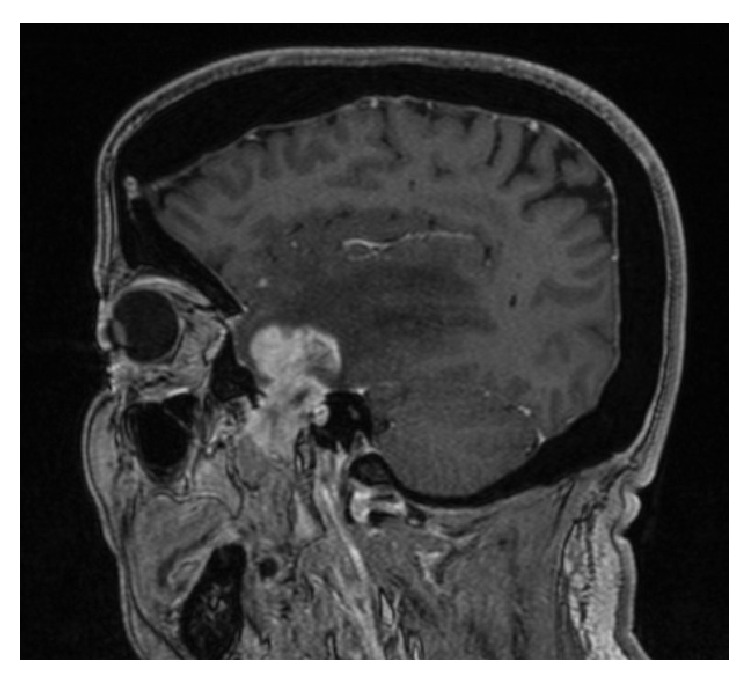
Preoperative enhanced parasagittal T1-WI MRI demonstrates extra-axial heterogeneously dumbbell-shaped enhancing tumor in the left middle fossa extending into infratemporal fossa.
